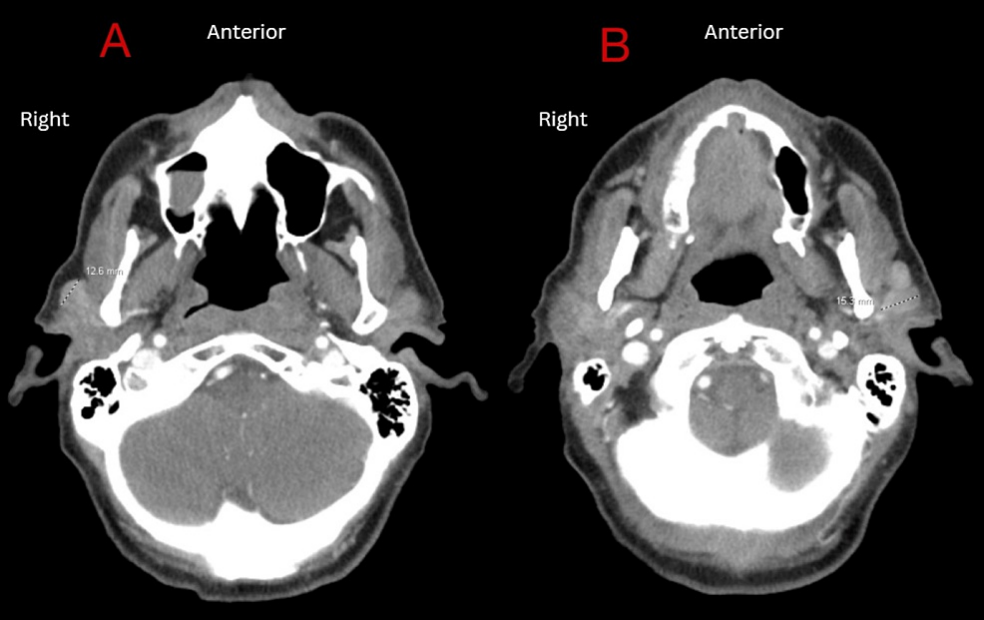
Head CT revealing a right 12.6mm (A) and a left 15.3mm (B) lymph node concerning lymphoma.
